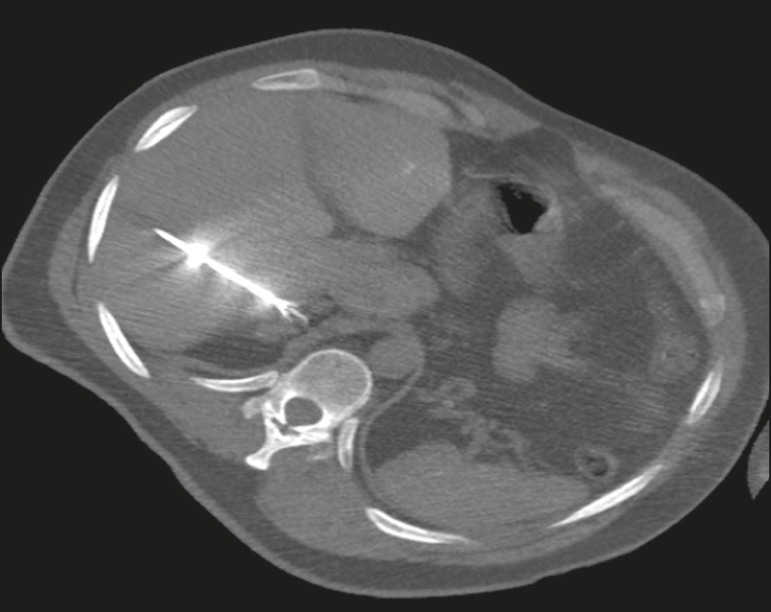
Axial computed tomography of the abdomen, showing percutaneous ablation
